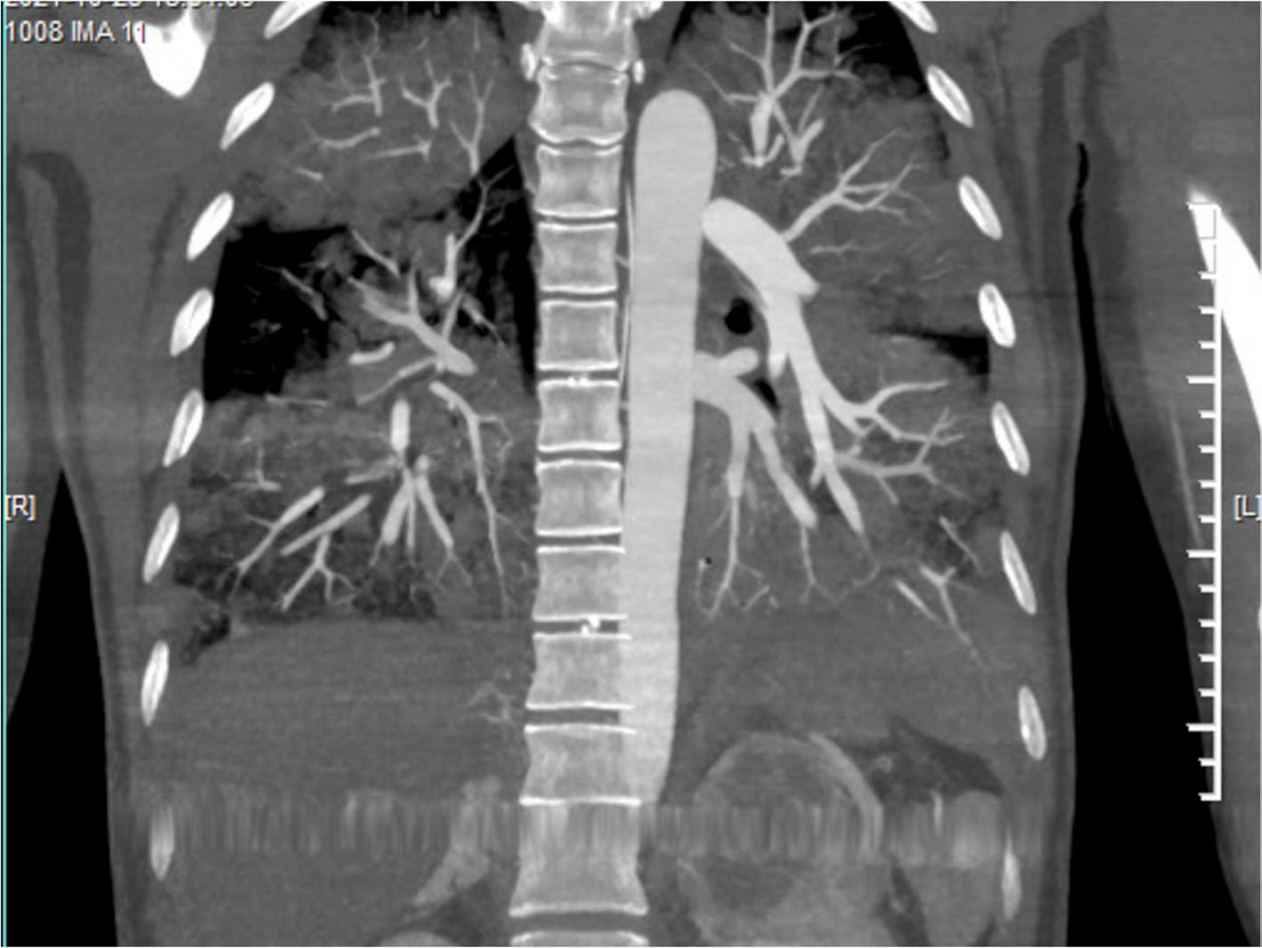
Chest CT showed bilateral diffuse infiltration
